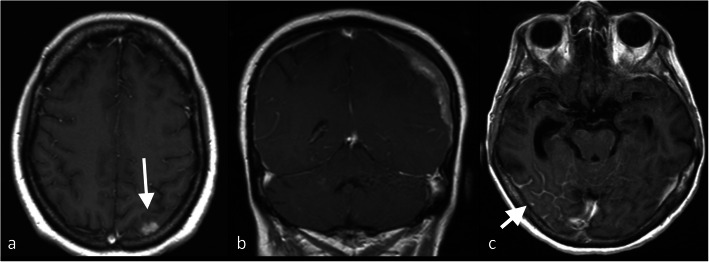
Cerebral manifestations of breast cancer. Post-contrast T1-W MRI in a 58-year-old woman with breast cancer demonstrates intracerebral metastases (a), dural metastasis (b) and leptomeningeal disease (c)
